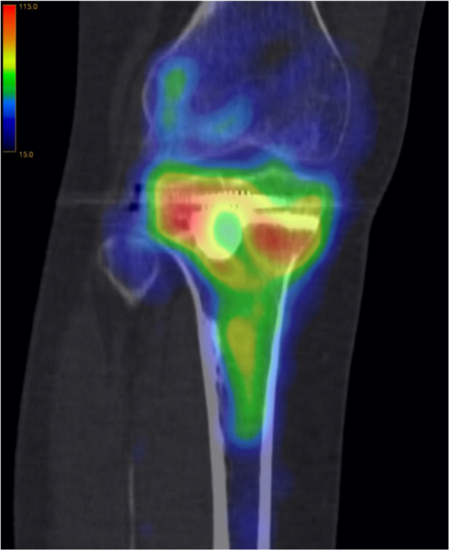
A coronal view of a SPECT/CT is shown 5 months after balloon tibioplasty. The blue to red colors show tracer activity in an increasing order and the normal to high tracer activity is particularly located around the cement and the osteosynthesis. In contrast, no color and no tracer activity can be found in the bone marrow of the tibial shaft
